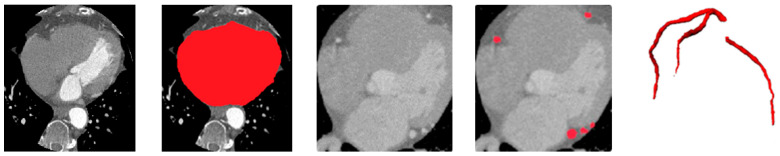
Qualitative results of heart and vessel segmentation. From left to right: original CT slice, heart segmentation, extracted heart ROI, coronary vessel segmentation, and 3D rendering of coronary vessels.
